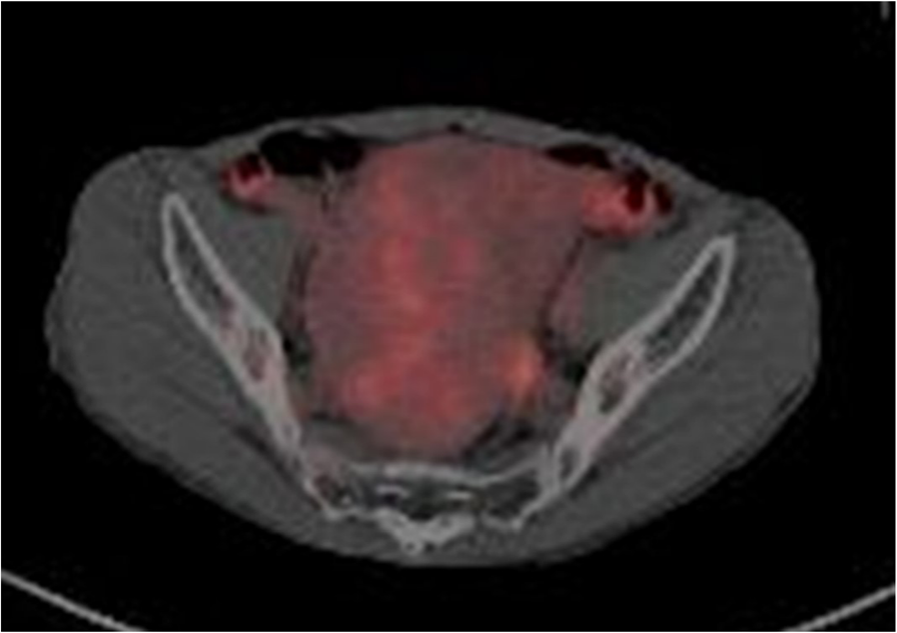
PET-CT image showing heterogeneous accumulation on the tumor. SUV-max level is 2.5
